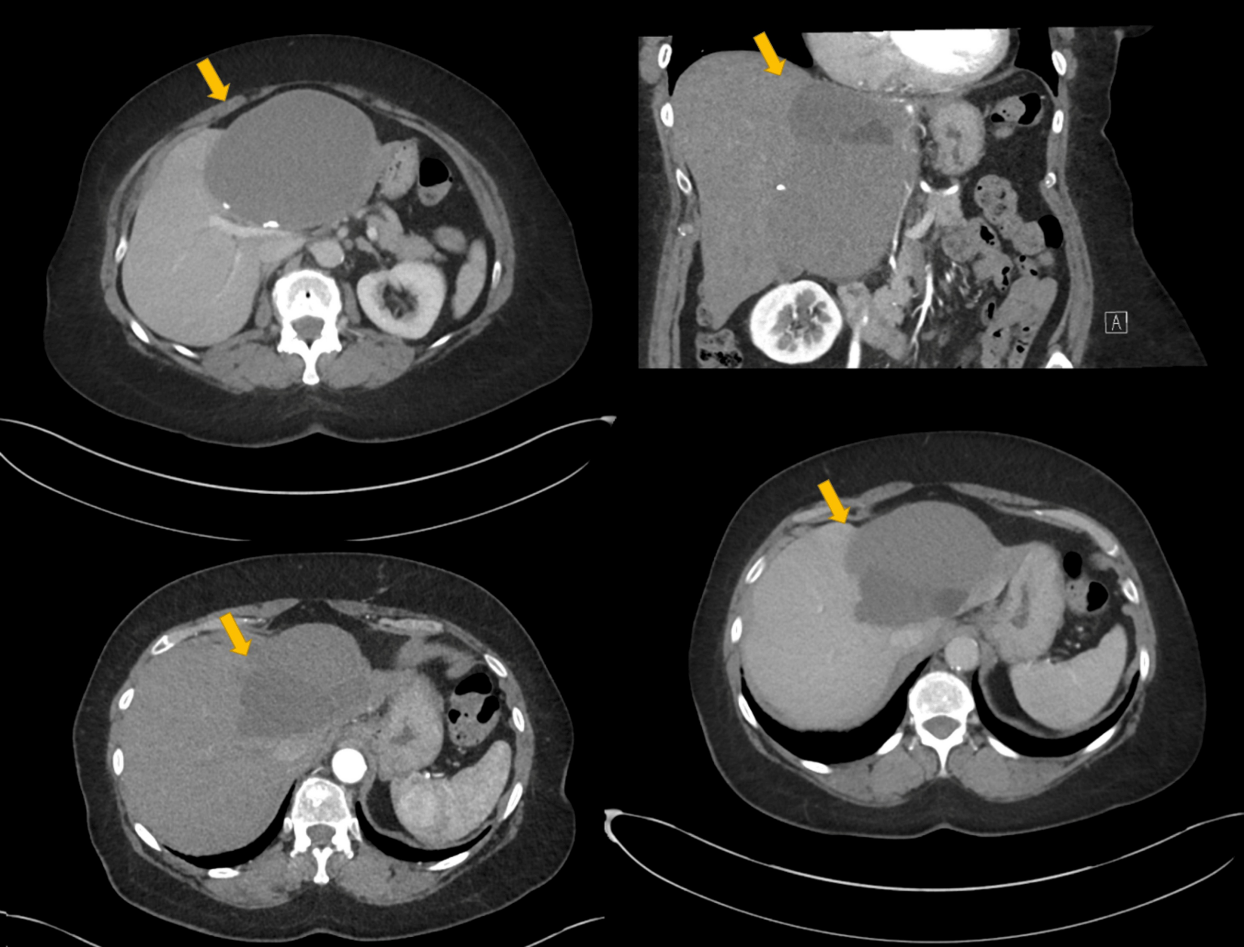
Axial and coronal CT images of BCAC. Axial (top left, bottom left, bottom right) and coronal (top right) contrast-enhanced CT images show a large, multiloculated cystic hepatic lesion (yellow arrows) with irregular wall thickening and subtle enhancing components. Internal septations and non-homogeneous fluid content are seen. These features were in keeping with malignant transformation, consistent with invasive BCAC. BCAC: biliary cystadenocarcinoma
